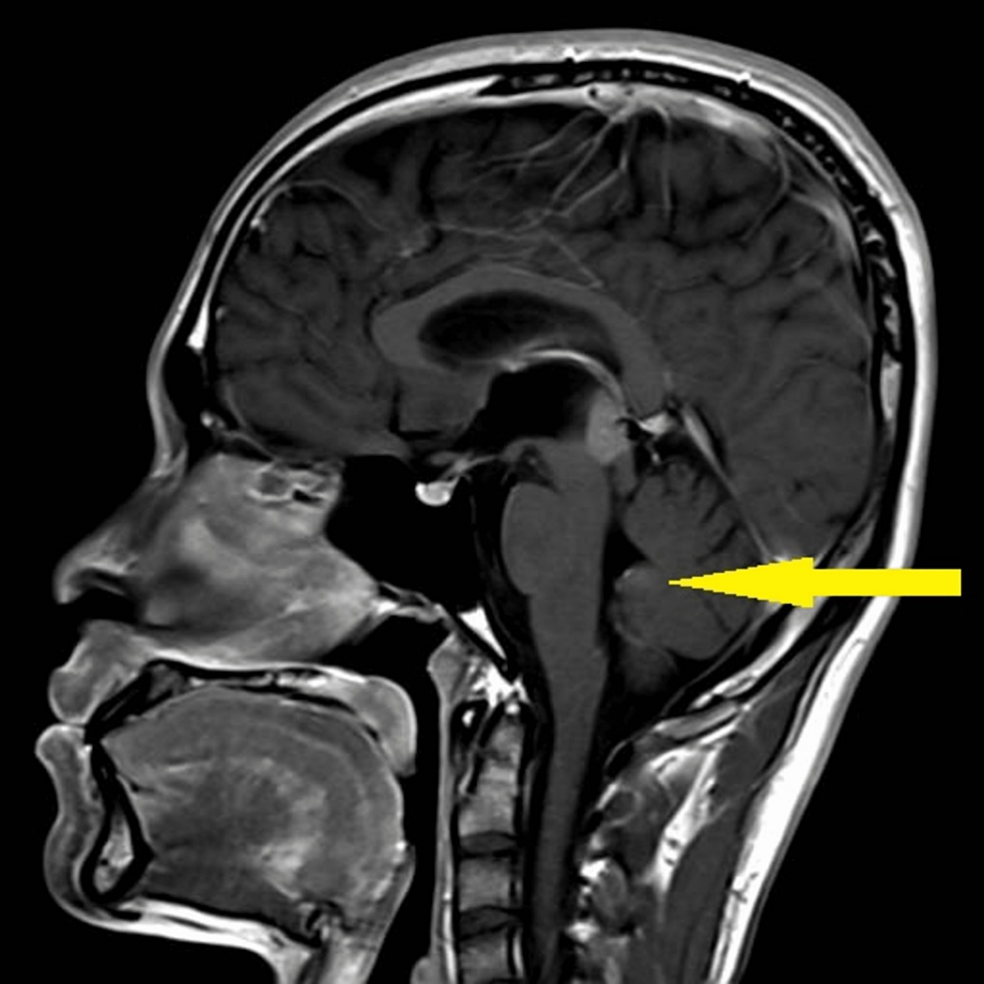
MRI brain (T1) sagittal showing an ill-defined lesion measuring 2.1 cm x 2.1 cm x 1.7 cm (yellow arrow)
